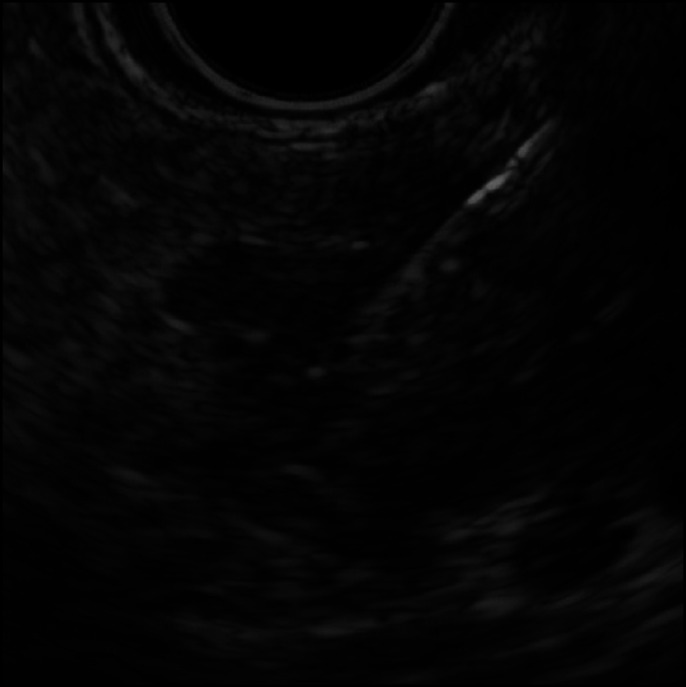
Endoscopic ultrasound-guided fine-needle aspiration performed only by one pass with a 19-gauge needle while carefully avoiding puncture of the pancreatic duct.
